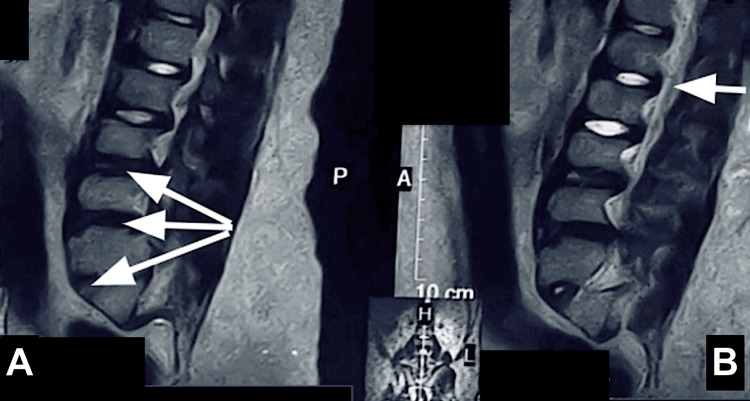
(A) Sagittal T2WI showing disc desiccatory changes at the L3-L4, L4-L5, and L5-S1 levels with reduced disc height at the L5-S1 level. (B) Sagittal T2WI showing a hypoplastic spinal cord. T2WIs: T2-weighted images
